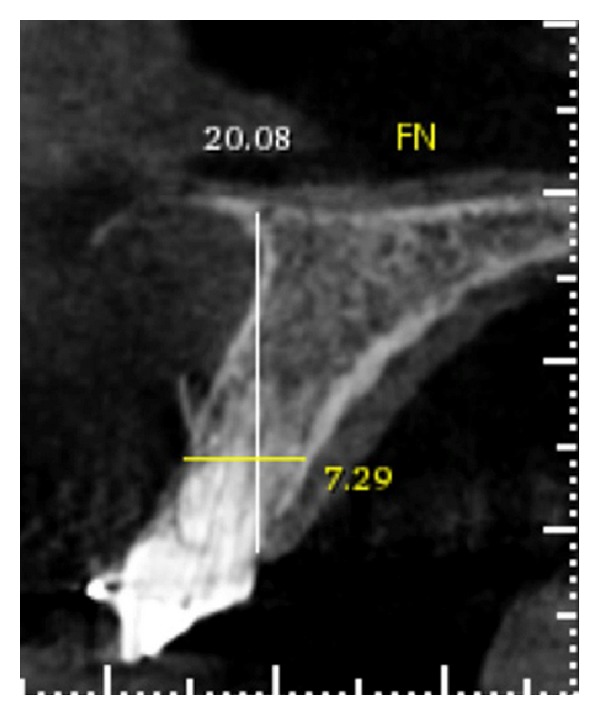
Computed tomography after orthodontic extrusion. Perforation was observed in the root.
